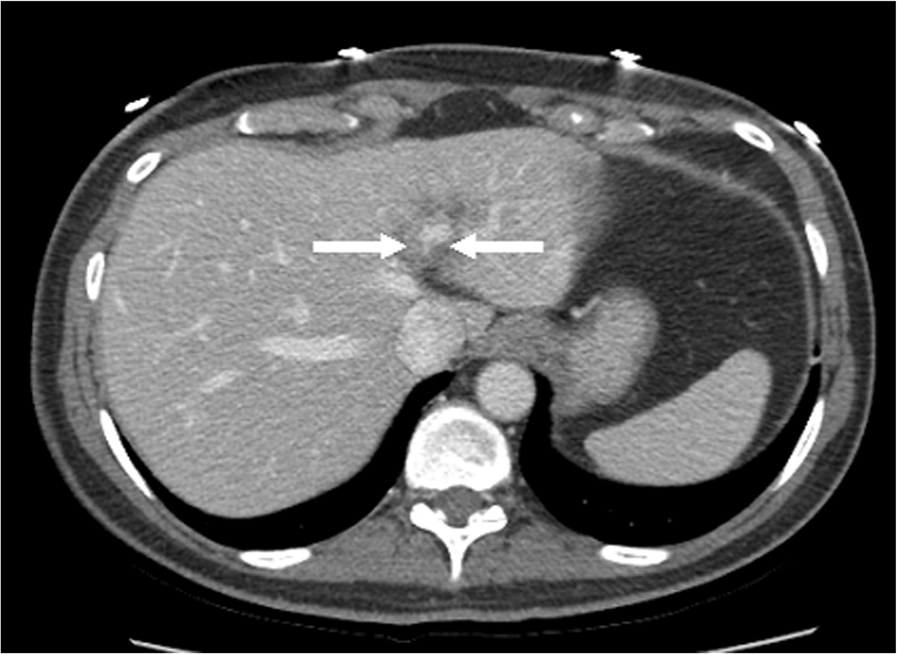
CT scan of the abdomen showing a dramatic decrease in size of the metastasis (marked by arrows) in the left lobe of the liver after treatment with eight cycles of pertuzumab, trastuzumab, and docetaxel.
